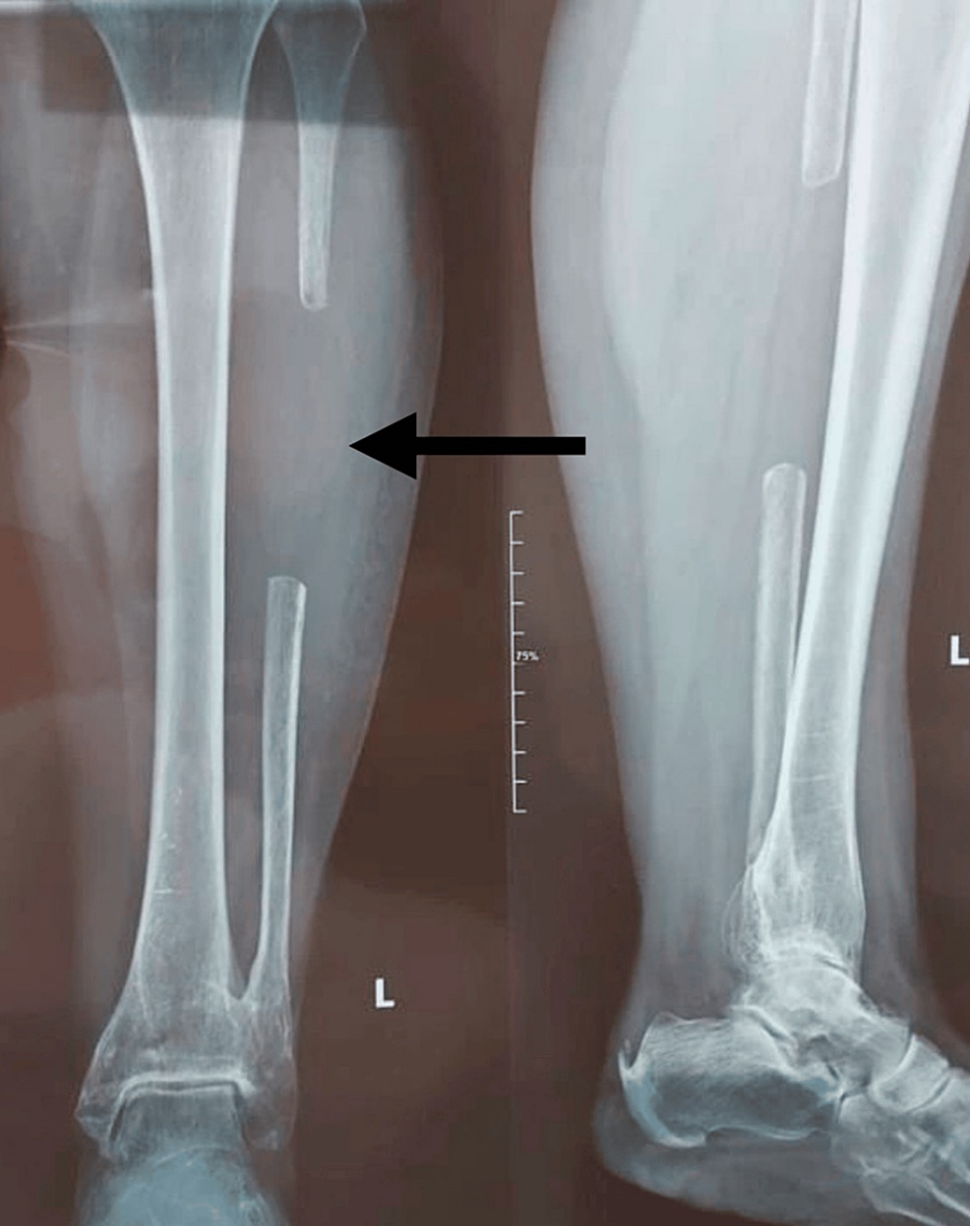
Postoperative AP and lateral X-ray of patient’s contralateral (left) leg showing fibular autograft donor site The arrow shows the donor site located in the shaft of the left fibula.
